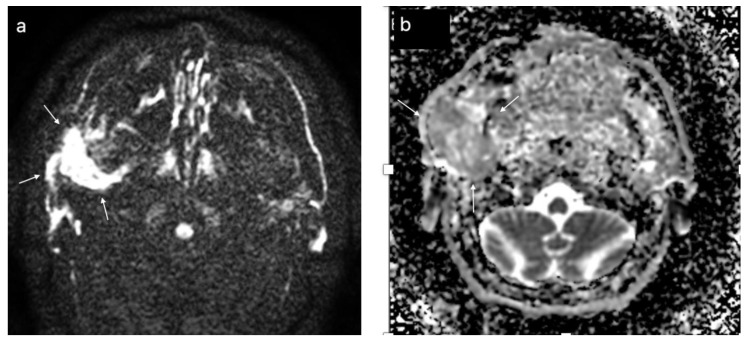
Malignant tumor of the left parotid gland (white arrows); DWI sequences (a) with ADC map (b) showing a value of 1.1 × 10^−^^3^ mm^2^/s.
